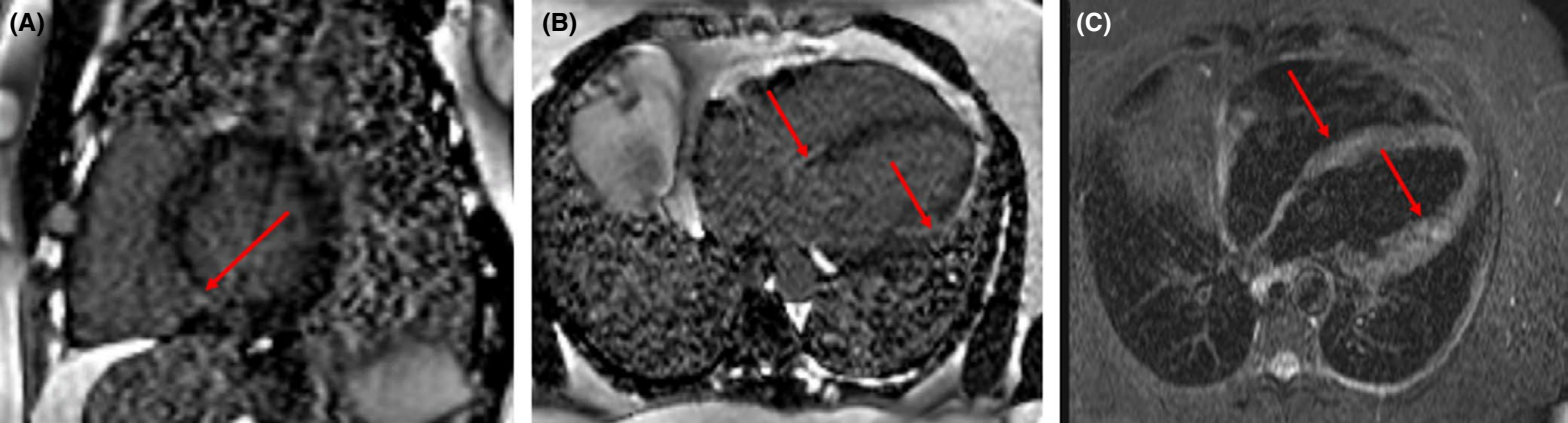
Cardiac MRI images showing late gadolinium enhancement of the mid‐septum A, basal to mid‐septum and lateral LV wall B, with corresponding edema and inflammation in STIR images C
